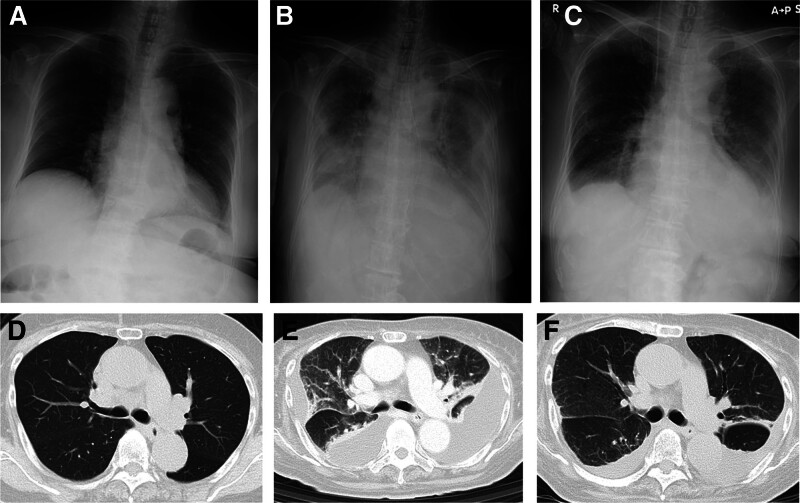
Chest X-P (1A) and chest CT (1D) on admission (day 1) showing no interstitial shadows or pleural effusion. Chest X-P (1B) and chest CT (1E) on day 70 after admission and before introduction of TCZ. Significant interstitial shadows and pleural effusion can be seen. At this point, the patient underwent weekly thoracic drainage due to increased pleural effusion over time and repeated hypoxemia. Chest X-P (1C) and chest CT (1F) post-TCZ induction (day 100) (chest X-P after day 2 of initiating TCZ). Interstitial shadows and pleural effusion are improved. CT = computed tomography, TCZ = tocilizumab.
